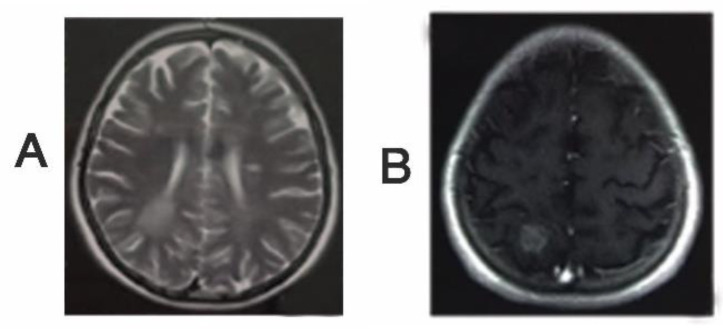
A, B: Brain MRI revealed high signal periventricular lesions. A tumefactive demyelinating lesion in right parietal lobe with surrounding vasogenic edema was also observed. There was post contrast enhancement of lesion
